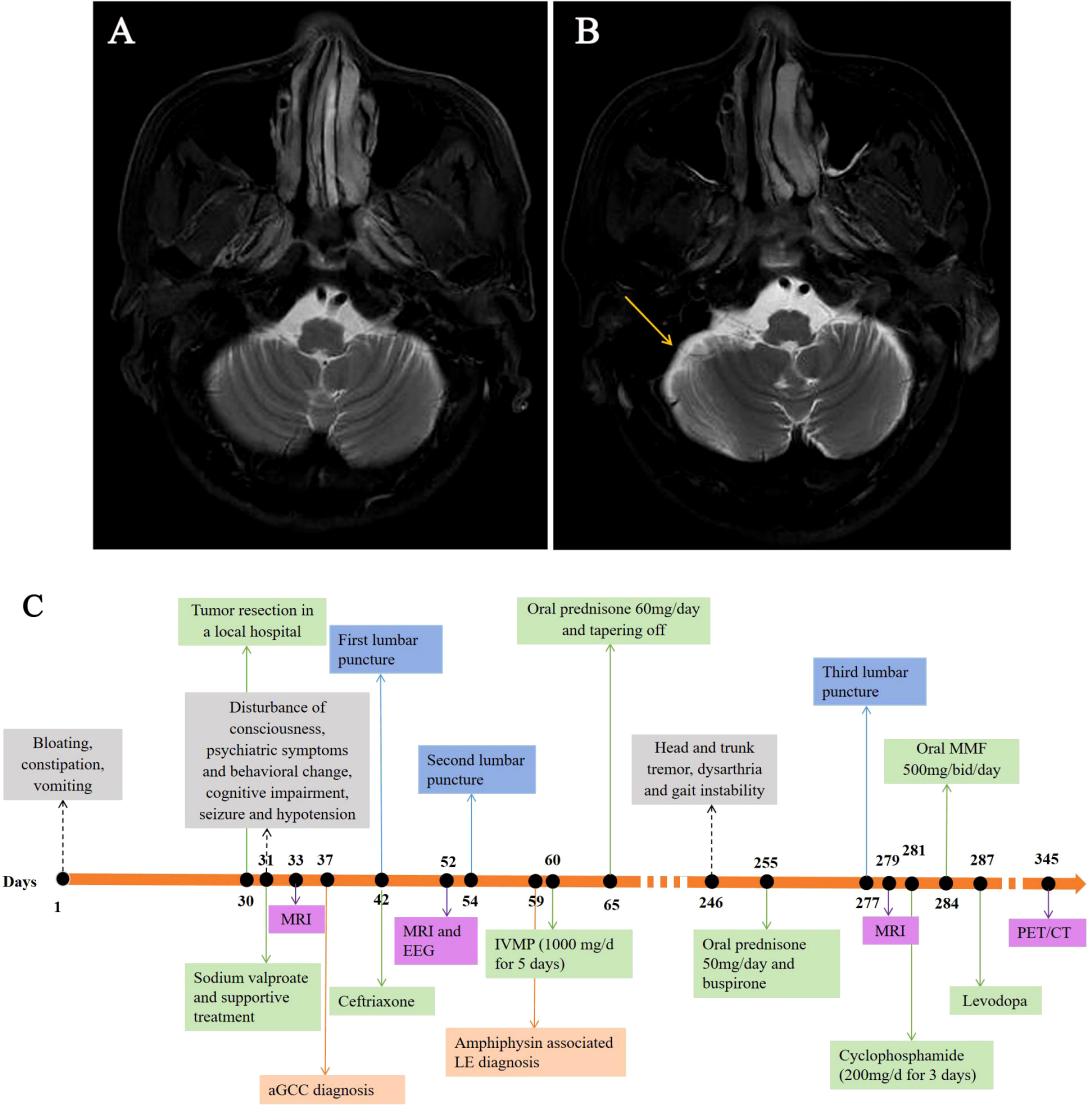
Brain MRI scans obtained from the patient and timeline of his clinical course. Despite there being no specific findings upon an initial T2-weighted MRI (A), mild cerebellar atrophy was observed in the subsequent axial T2-weighted MRI performed seven months later (B, yellow arrow). Timeline of the patient’s clinical course highlighting the manifestation, auxiliary examination, treatment and diagnosis time (C). EEG, electroencephalograph; LE, limbic encephalitis; MMF, mycophenolate mofetil; MRI, magnetic resonance imaging; IVMP, intravenous methylprednisolone; PET/CT, positron emission tomography with computed tomography.
